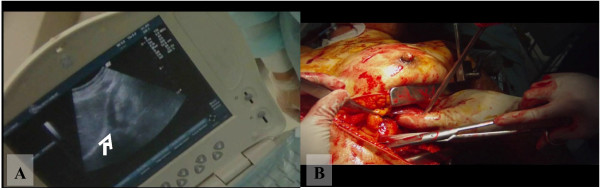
Emergency thoracotomy in the emergency room with pericardium incision. A: Subxiphoid view of bedside echocardiography performed by surgeon equipped with a head-cam. The presence of pericardial fluid (white arrow) enabled the diagnosis of cardiac tamponade to be made (video image capture). B: Left anterior thoracotomy and longitudinal incision over the pericardium made in the emergency department. The image shows significant hemorrhaging from the pericardium (video image capture).
